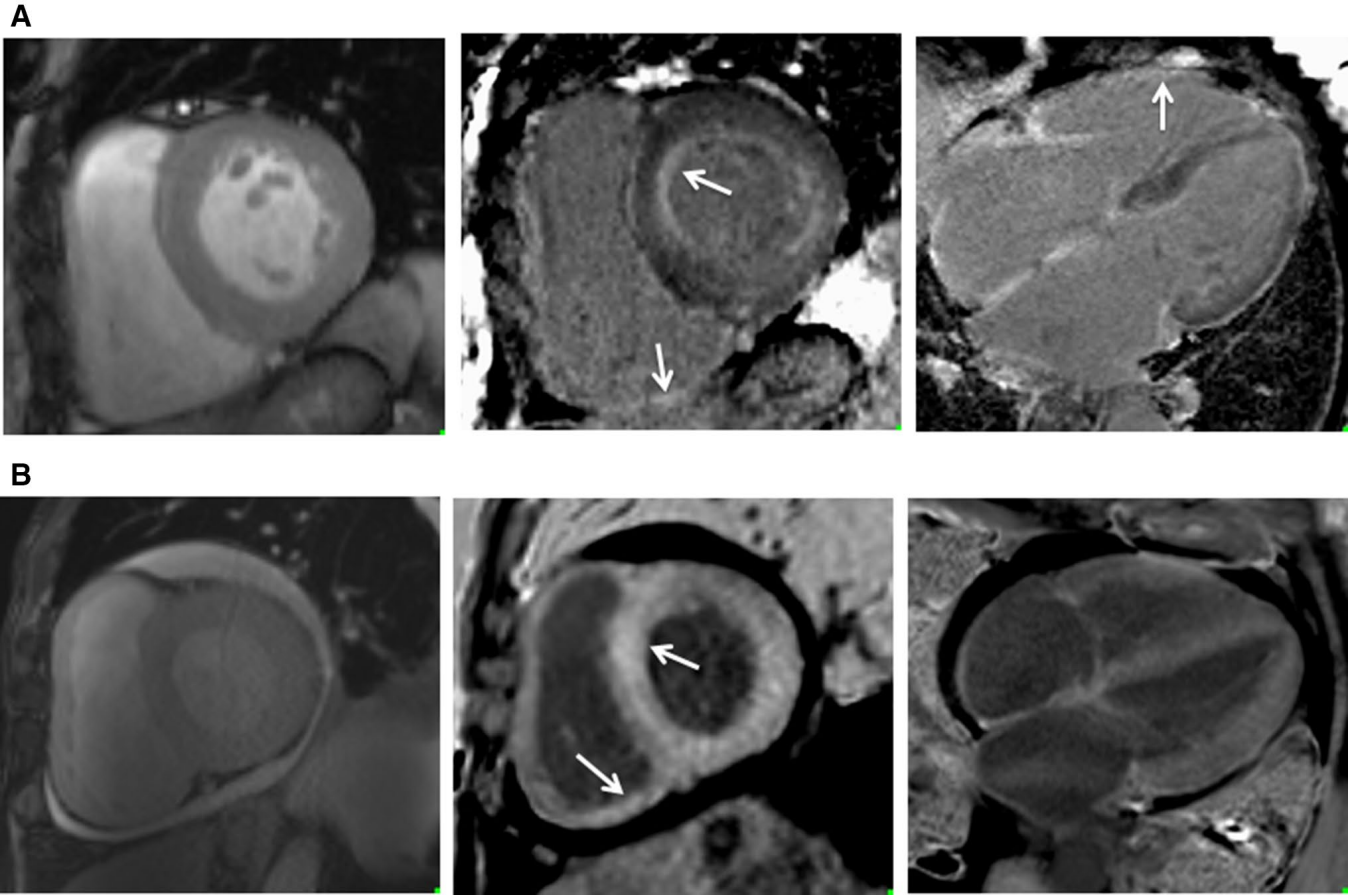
Representative two cases with cardiac amyloidosis. a A 68-year-old female with light chain amyloidosis. CMR cine in the short-axis view demonstrates LV hypertrophy. Cine-MR showed preserved normal biventricular systolic global LV function (LVESVi 33.5 mL/m^2^, LVEF 51.0%, RVESVi 35.5 mL/m^2^, RVEF 62.3%). The LGE-MRI images on the mid and right panel showed subendocardial LGE in the left ventricle and LGE in the right ventricle (*arrow* indicated). The patient did well during follow-up. b A 76-year-old male patient with reduced RVEF. CMR cine in the short-axis view demonstrates biventricular hypertrophy, increased right ventricular wall thickness, and circumferential pericardial effusion. LGE positive was shown in LV and RV (*mid*-*panel*, *arrows*). Cine-MR showed altered systolic global LV function (LVESVi 59.9 mL/m^2^, LVEF 31.0%, RVESVi 65.2 mL/m^2^, RVEF 34.0%). Despite optimal medical therapy, this patient suffered NYHA Class III heart failure and died in hospital after diagnosis with amyloidosis at the 6th month
